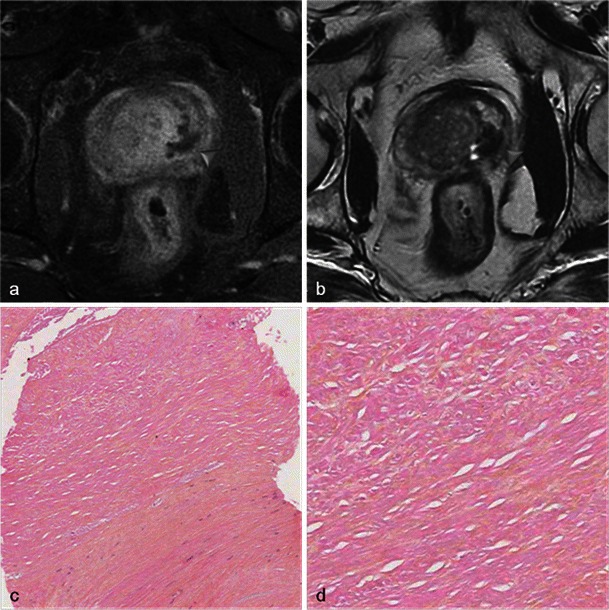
Residual necrosis. Six-month post-treatment MRI in a patient treated by left photodynamic therapy. Axial T1 FS contrast-enhanced (a) and axial T2-weighted images (b) show persistence of a low signal intensity area with no enhancement (*arrowhead*) corresponding to a small area of residual necrosis (coagulation necrosis). Pathology slides from biopsy at low (c) and high magnification (d) show loose connective tissue coreless, with cell ghosts, characteristic of coagulation necrosis
